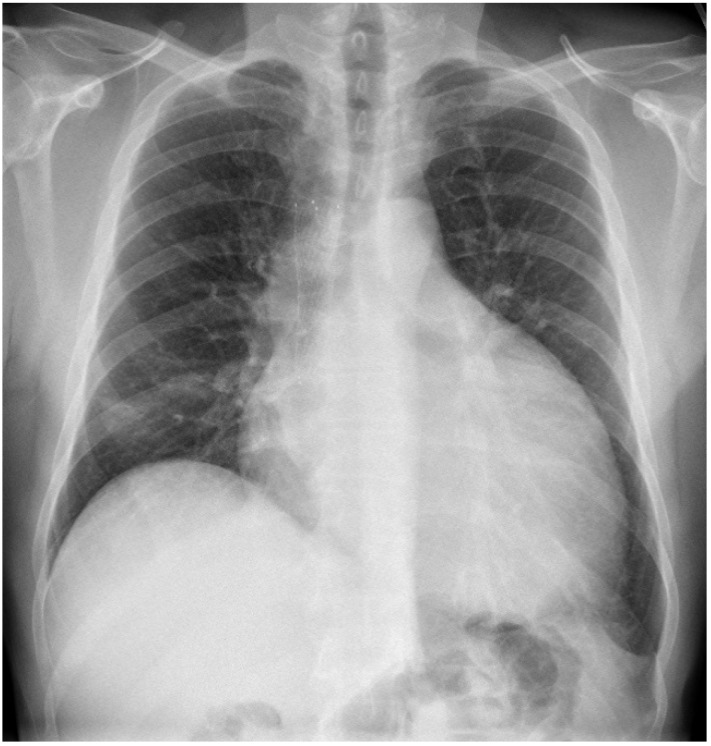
Chest X-ray. Enlargement of pericardial sillouette.
